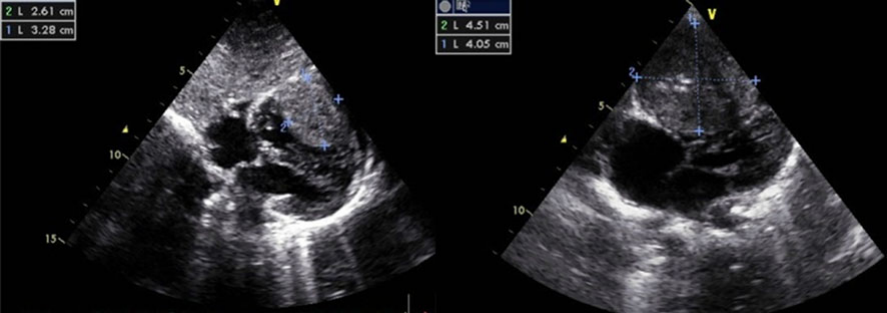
Transthoracic echocardiography showing a large homogeneous mass originating from the right ventricular free wall
